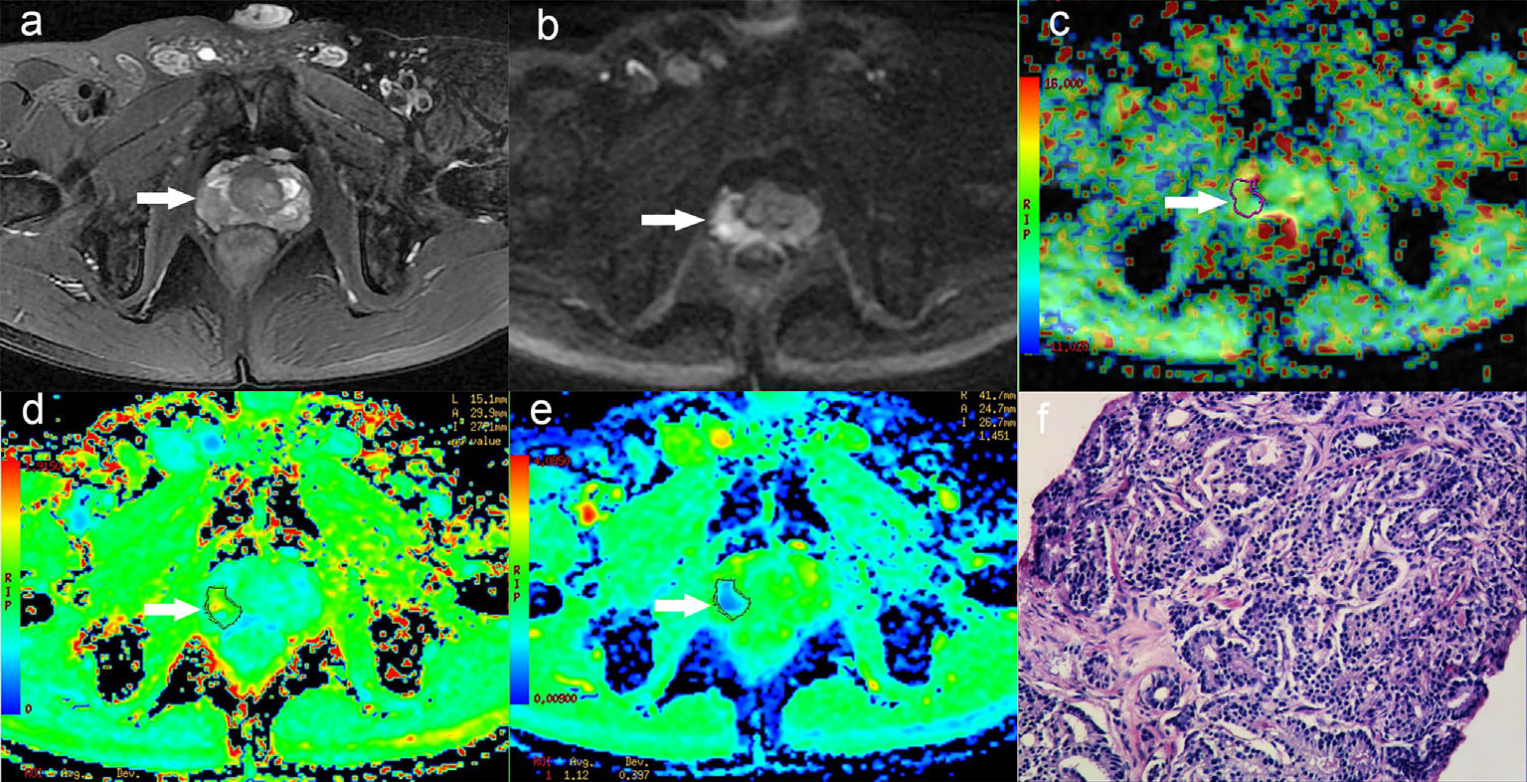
A 58-year-old man with PCa in right peripheral zone, GS is 8. (A) T2-weighted image shows hypointense signal in the lesion, (B) Diffusion-weighted imaging indicates hyperintense signal, (C) APT pseudo-colored map shows yellow-green pseudocolor in the lesion, (D) Mean kurtosis pseudo-colored map indicates red-yellow-green pseudocolor in the lesion, (E) Mean diffusivity pseudo-colored map shows blue-green pseudocolor in the lesion, (F) pathological image.
